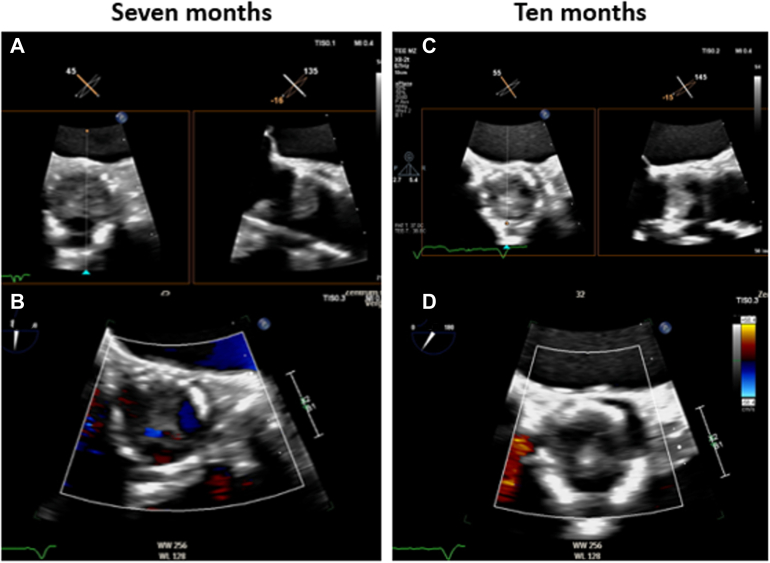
Case 2: Transesophageal Echocardiography at 7- and 10-Month Follow-Up (A and B) TEE at the 7-month follow-up during investigation for bacteremia, demonstrating subtotal valve thrombosis with minimal residual central forward flow. (C and D) TEE at the 10-month follow-up confirming complete thrombotic sealing with no transvalvular flow. TEE = transesophageal echocardiography.
